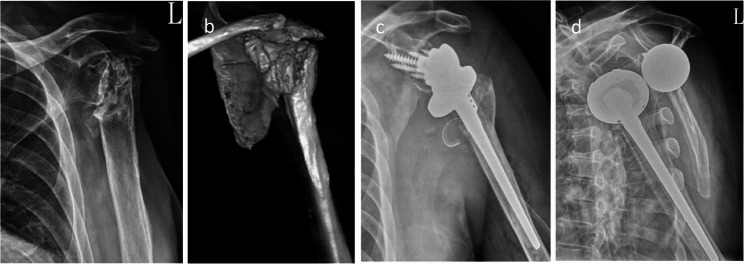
Case 2. a, b X-ray and CT scan of the proximal humerus fracture’s sequelae in a 69-year-old man. c RTSA at first time. d Dislocation after RTSA 2 weeks post-surgery
